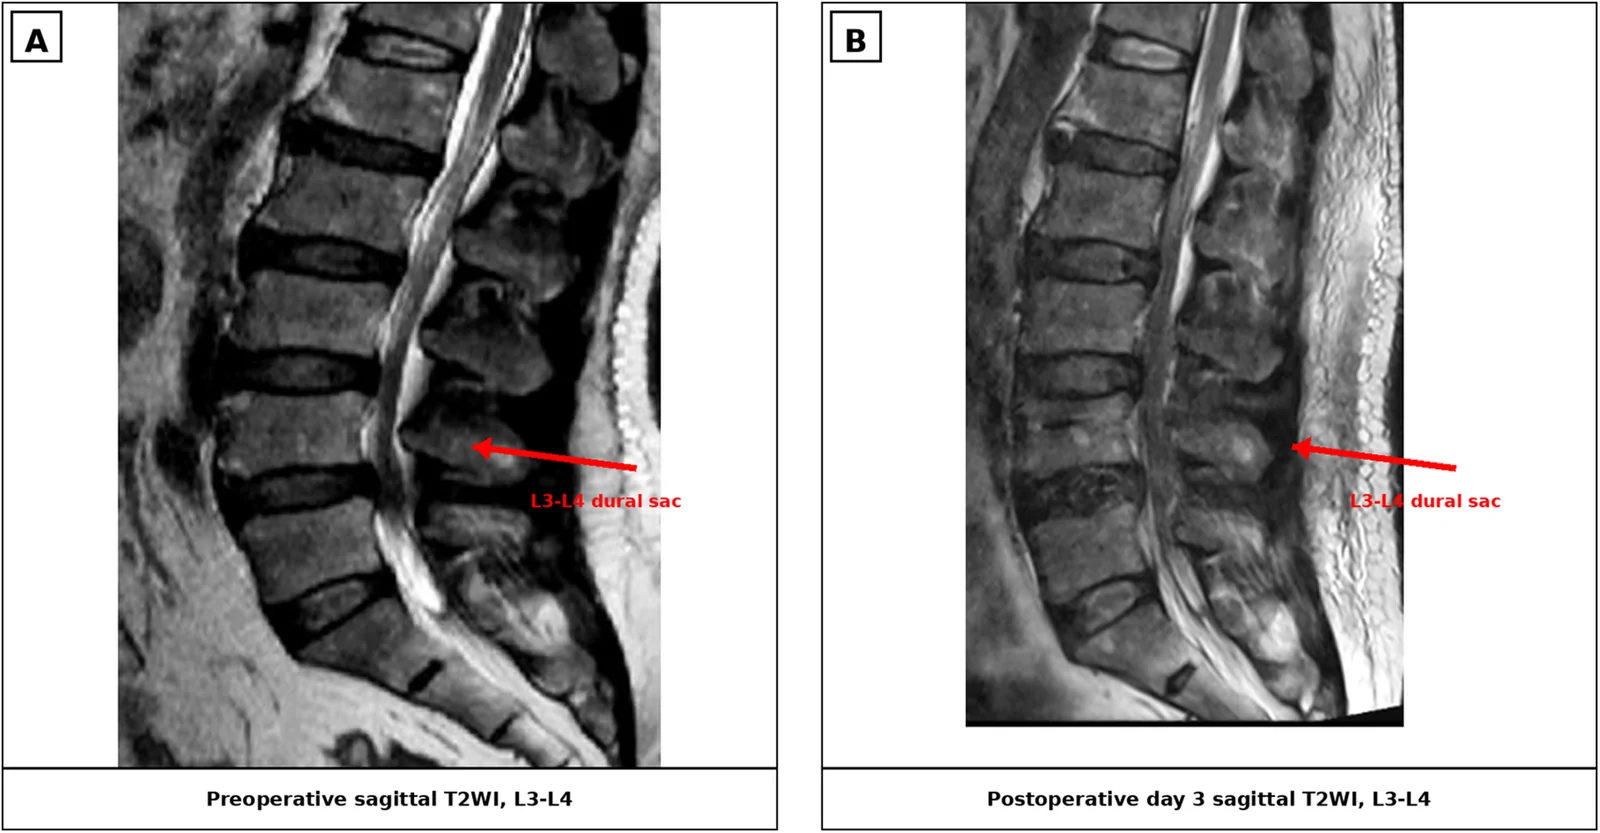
Direct preoperative and postoperative sagittal T2-weighted MRI comparison at L3-L4. (A) Preoperative sagittal T2-weighted MRI showing posterior indentation and compression of the dural sac at L3-L4. (B) Sagittal T2-weighted MRI obtained on postoperative day 3 in the closest comparable anatomical plane, showing reduced posterior indentation of the dural sac and increased visible cerebrospinal fluid space at L3-L4. The level and relevant dural sac region are marked consistently in both panels.
